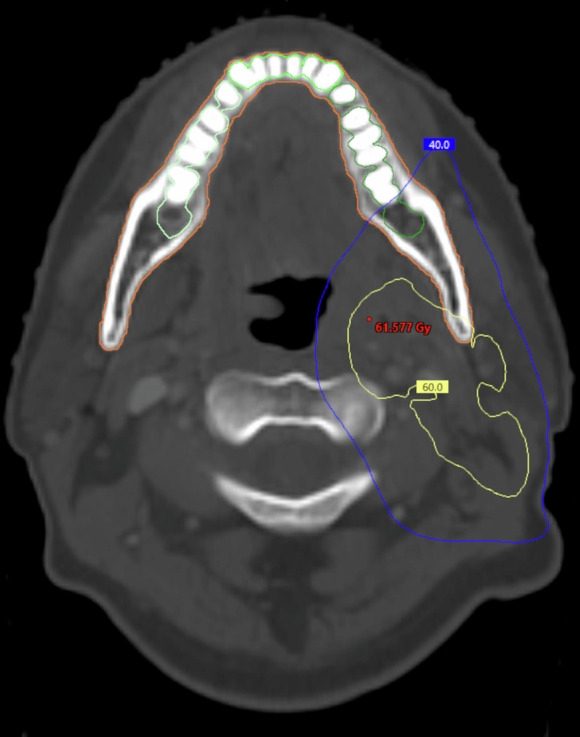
CT scan of patient with oral cavity cancer who received ipsilateral RT with 66 Gy. The figure shows that 40 Gy threshold barely reaches even the molars.
